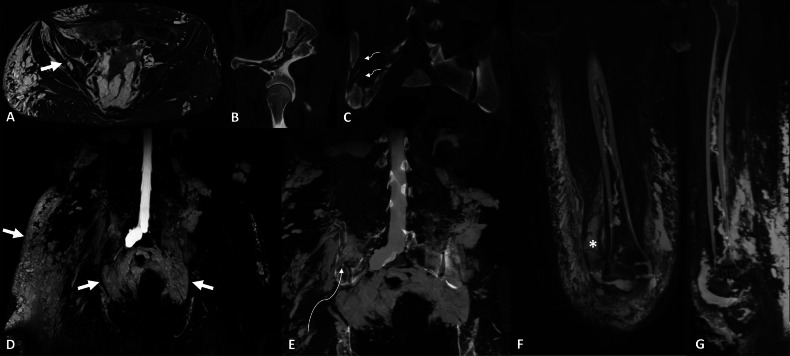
Work-up for recurrent septic arthritis of the right knee and right buttock swelling in a 35-year-old male. A STIR, axial view. Linear snaking lesion (arrow) of the right iliac bone with high signal intensity on T2w images. B, C Noncontrast CT, sagittal oblique (B) and coronal (C) views. Corresponding anomalies with low attenuation density. The “point of entry” of the lymphangiectasis (curved arrows) in the iliac bone is well identified on the coronal view. D 3D FRFSE T2w, MIP reconstruction (20 mm), coronal view. NEMRL reveals diffuse lymphatic anomalies with pelvic and subcutaneous lymphangiectasis (arrows). These findings are compatible with diffuse primary lymphangiectasis. E Fusion of NEMRL and CT images, coronal view. NEMRL brings to light a connection between the pelvic lymphangiectasis and intraosseous lymphangiectasis (curved arrow) through the point of entry identified on CT. F, G NEMRL of the lower extremities reveals intraosseous lymphangiectasis of the femoral diaphysis and of surrounding soft tissues. Fluid effusion is also identified (*). Impaired lymphatic drainage of the right lower extremity is most likely responsible for the recurring septic arthritis of the knee
